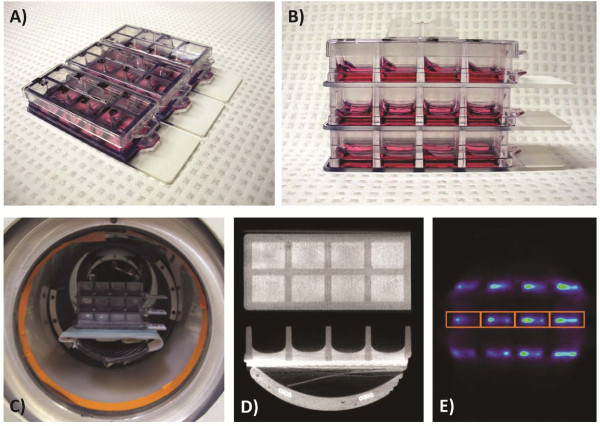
A) Cells seeded in chamber slides, B) Stacked chamber slides for PET scanning, C) Aligning stack in PET axial and trans-axial field of view (FOV), D) CT images used to draw regions of interest (ROI), E) PET image with an example of projected CT-defined ROI for PET data analysis.
